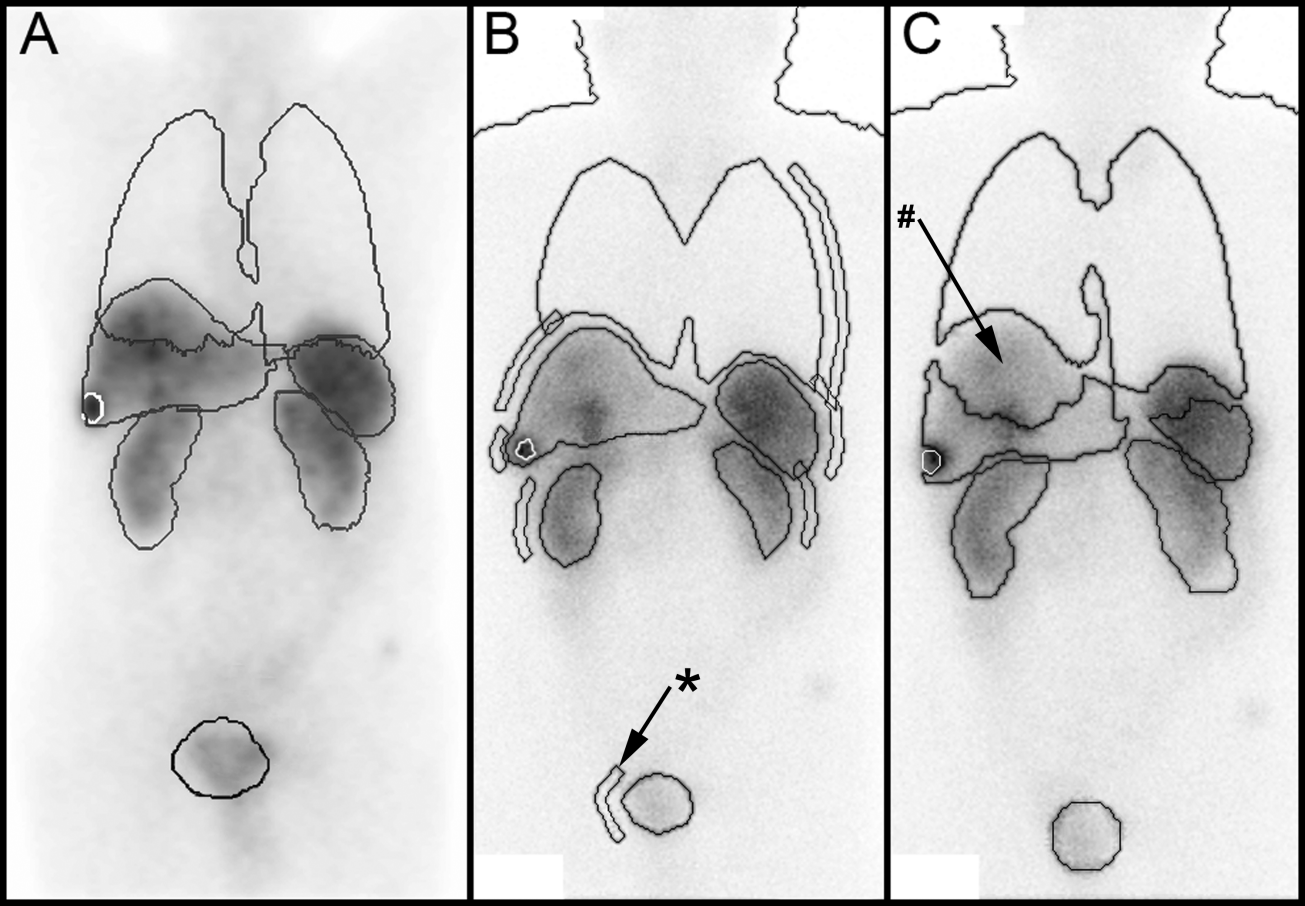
ROI/VOI comparison of all three imaging scenarios 24 h p.i. of the same patient. The delineations of lungs, liver, kidneys, spleen, bladder and tumour (white, in the liver) are shown. (A) 2D presentation (summed coronal slice) of the 3D VOIs of the multi-SPECT-CT scenario. (B) Geometric mean image of the planar scenario with all ROIs. The small elongated delineations (*) next to the ROIs were used for background correction. (C) Geometric mean image of the WB scintigraphies with SPECT/CT based VOIs (hybrid scenario). Here, overlapping regions of interest were automatically removed (#) and corrected.
